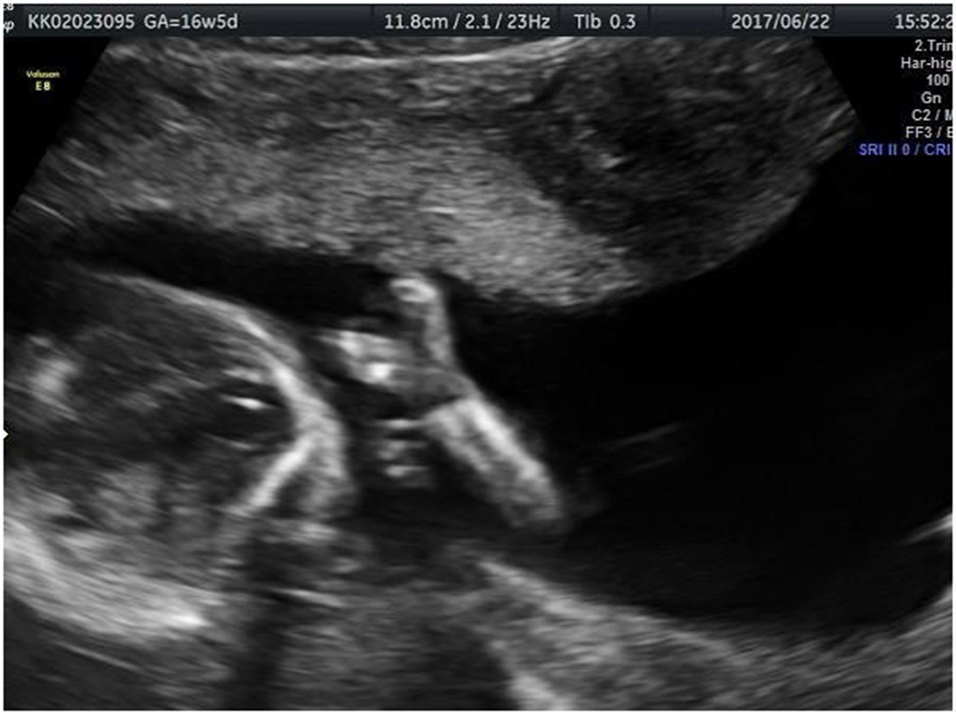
EEC syndrome. Two-dimensional ultrasound demonstrates the lobster-claw hand.
